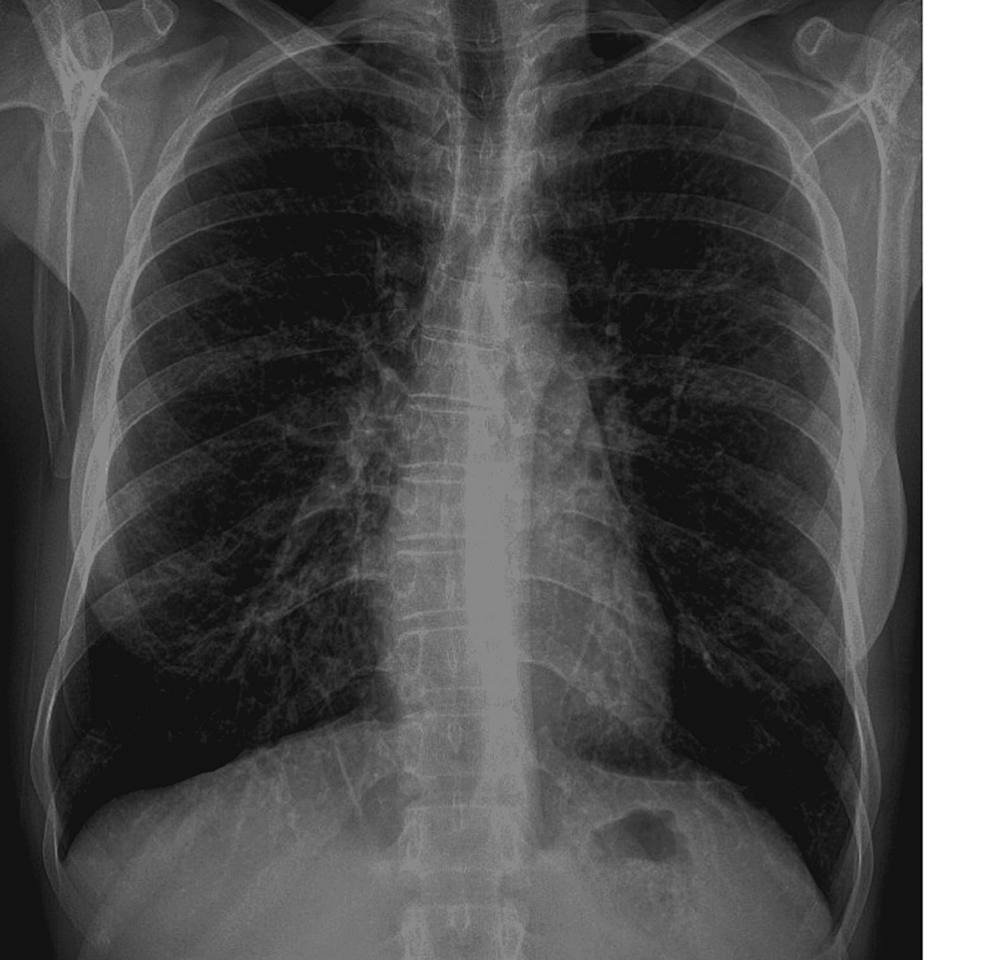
Follow-up chest radiograph after two weeks showing complete resolution of radiological abnormalities (normalized).
